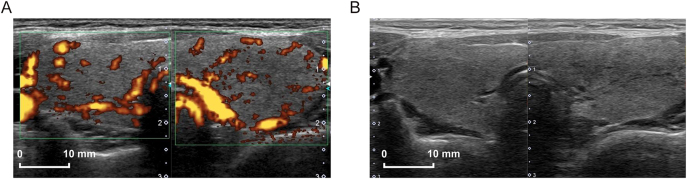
Representative thyroid ultrasound images. (A) Doppler ultrasound at 11 weeks of gestation during the first pregnancy shows diffuse thyroid enlargement with increased vascularity. The right lobe measured 22.1 × 15.9 mm, and the left lobe measured 25.3 × 16.8 mm. (B) Thyroid ultrasound at nine weeks of gestation during the fourth pregnancy demonstrates a heterogeneous parenchyma without significantly increased blood flow. The right lobe measured 21.6 × 15.7 mm, and the left lobe measured 25.7 × 16.0 mm. The scale bar represents 10 mm.
